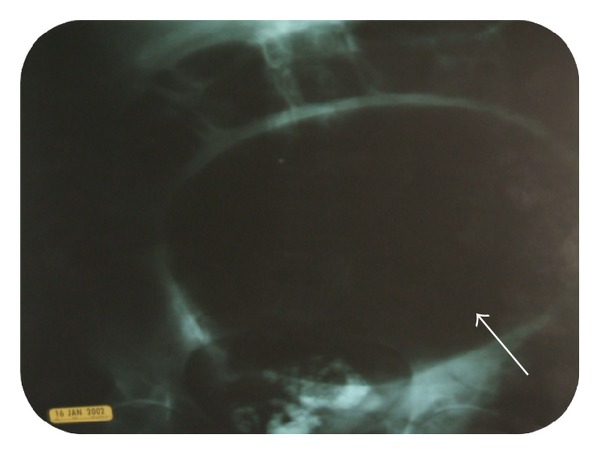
Abdominal X-ray showing air fluid level with a dilated loop of sigmoid colon.
